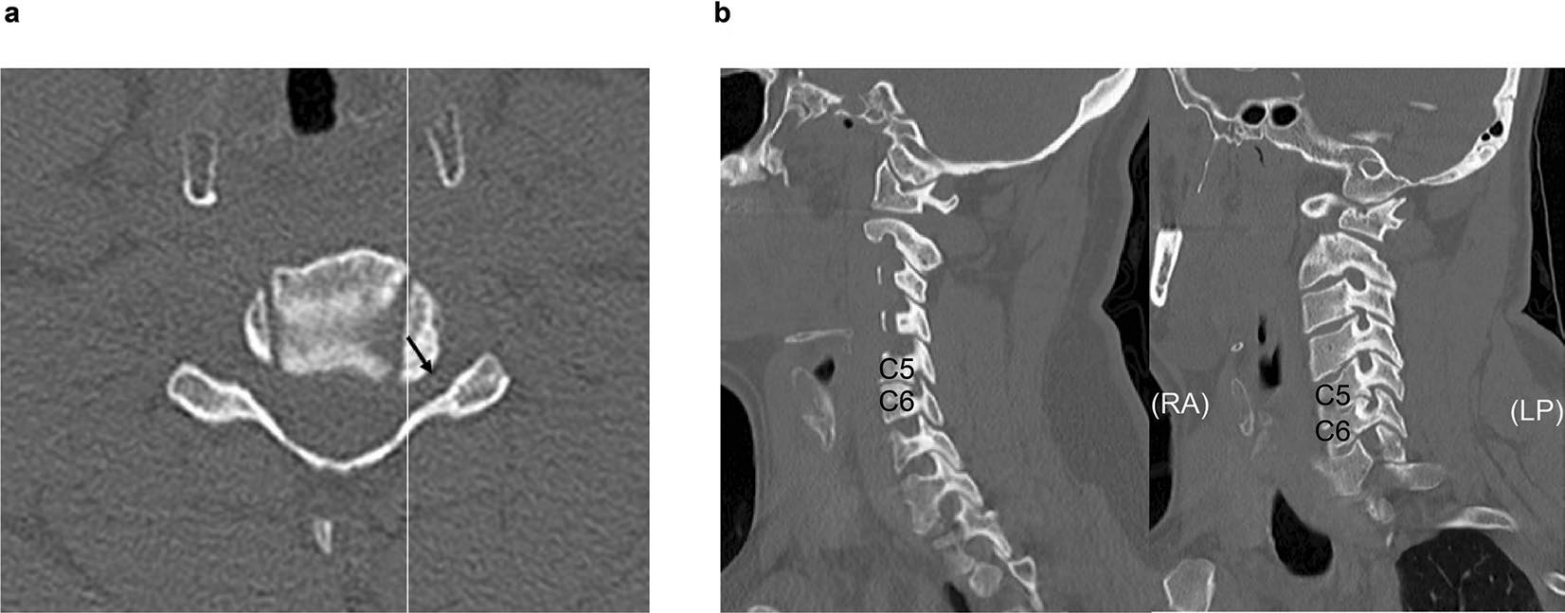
(a) Axial CT at C5/6: Line is a sagittal slice direction; arrow is an osteophyte direction. (b) Left: Foraminal narrowing at C5/6 in normal sagittal CT; Right: Foraminal narrowing at C5/6 in oblique reformatted sagittal CT. Both were observed in the same patient, but the normal sagittal view showed less foraminal narrowing.
